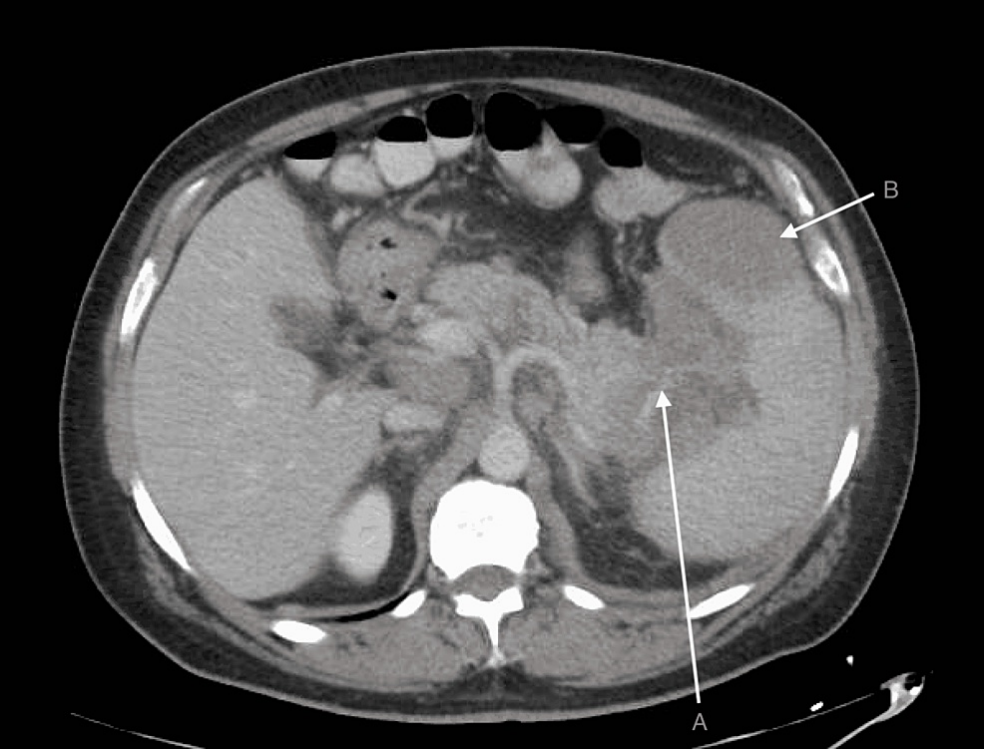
CT of the abdomen and pelvis demonstrating a large hypodense mass in the tail of the pancreas and a well-demarcated hypodense lesion in the anterior pole of the spleen A: Mass involving the tail of the pancreas extending and invading the splenic hilum. B: Mass within the anterior aspect of the spleen.
